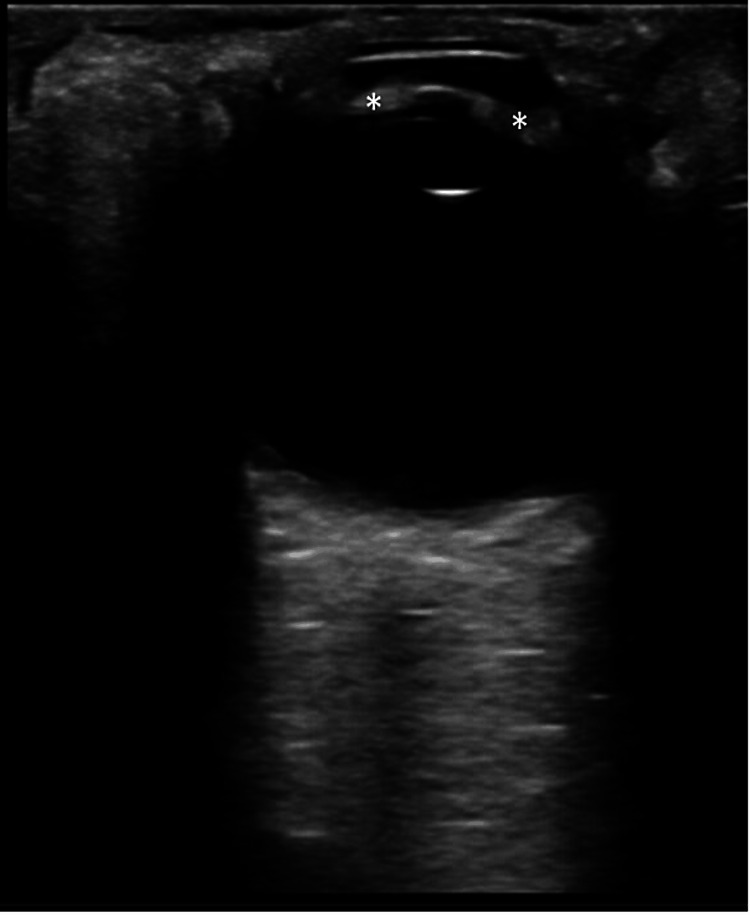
Point of care ocular ultrasound Ocular ultrasound showing the uvea(*), which appears thickened
